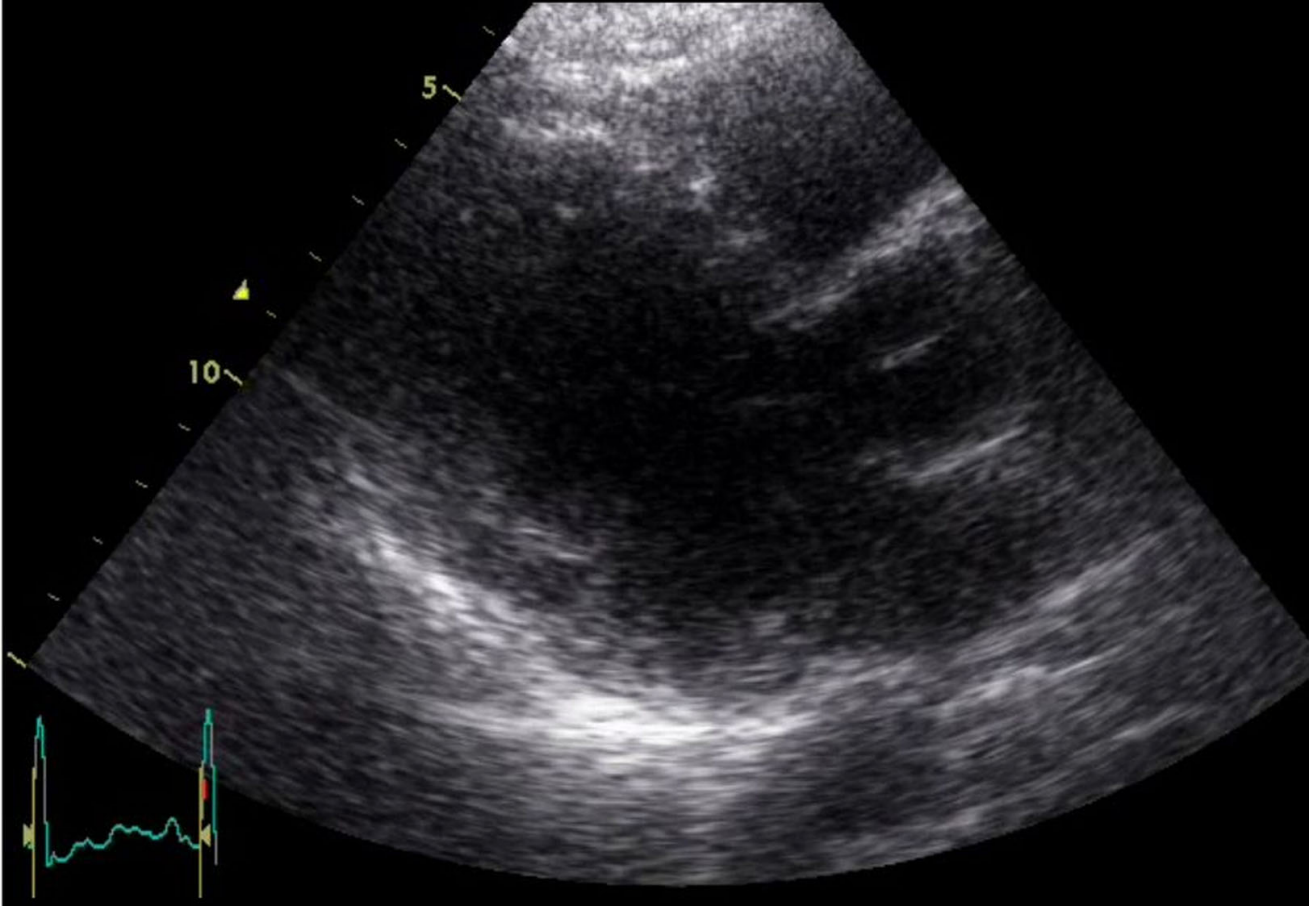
Follow up transthoracic echocardiography. Parasternal long axis view six months after the operation depicting a normal LV cavity.
